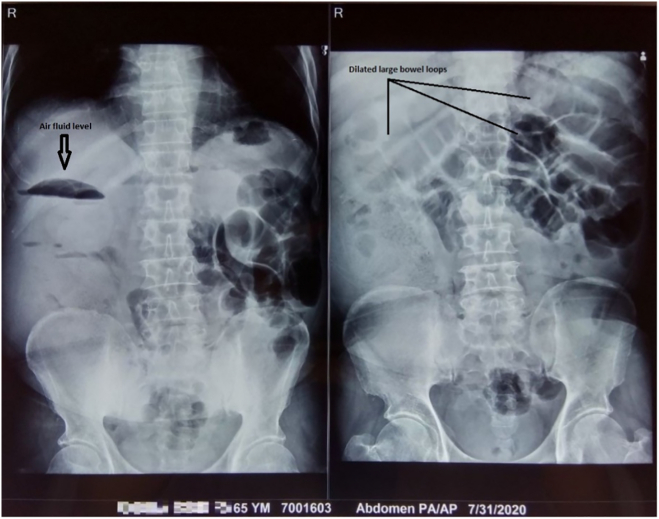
Erect abdominal X-ray showing dilated large bowel loops and air fluid level.
